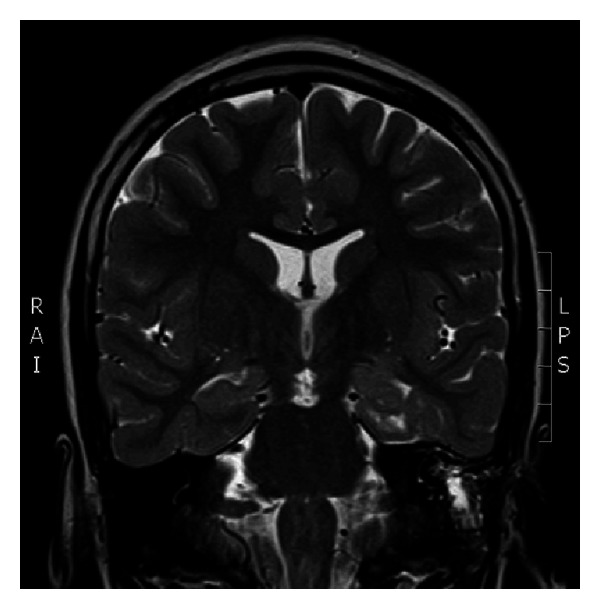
Coronal T2-WI MRI scan demonstrating the left mesial temporal lesion.
